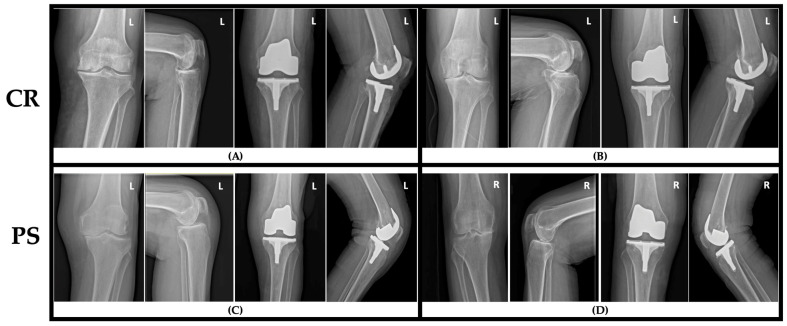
Panels (CR, A) and (CR, B) show two different patients treated with a cruciate-retaining implant, with representative anteroposterior and lateral radiographs obtained preoperatively and at 24 h postoperatively. Panels (PS, C) and (PS, D) show two different patients who underwent posterior-stabilized TKA with perioperative anteroposterior and lateral views. The images show the typical radiographic appearance and component positioning associated with CR and PS designs from Smith & Nephew Legion Genesis II implant system.
